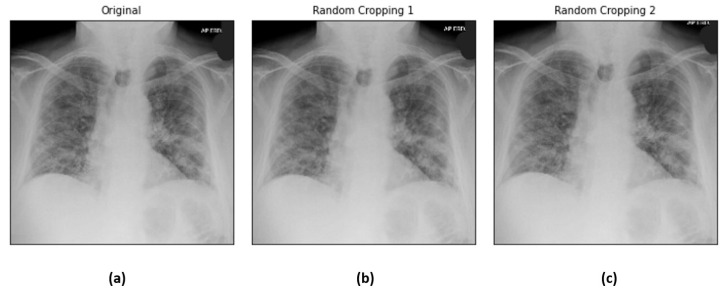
Sample chest X-ray images: (a) original, (b) random cropping (r−5,c−5) and (c) random cropping (r−10,c−10).
